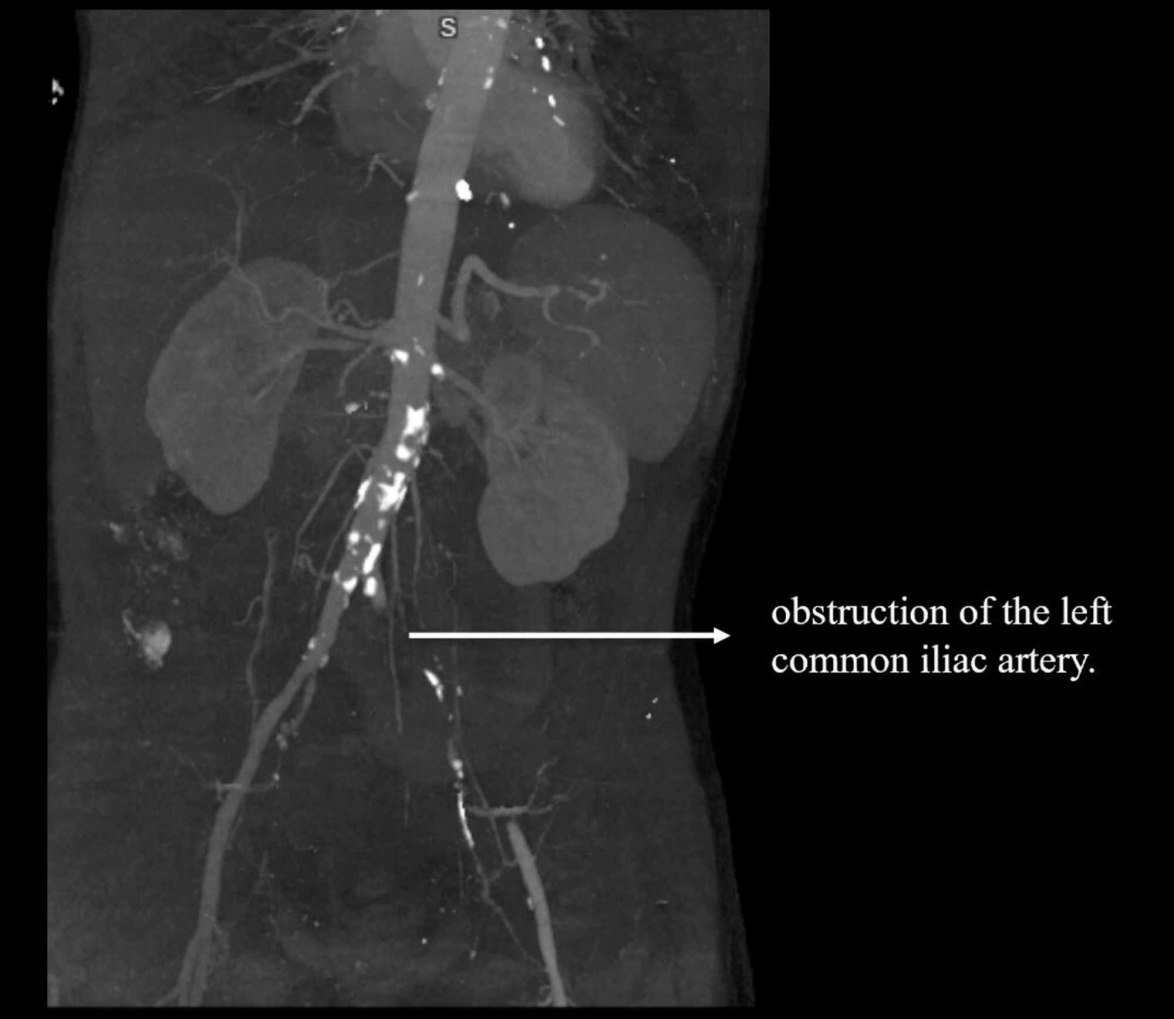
CT maximum intensity projection (MIP) demonstrating occlusion of the left common iliac artery.
